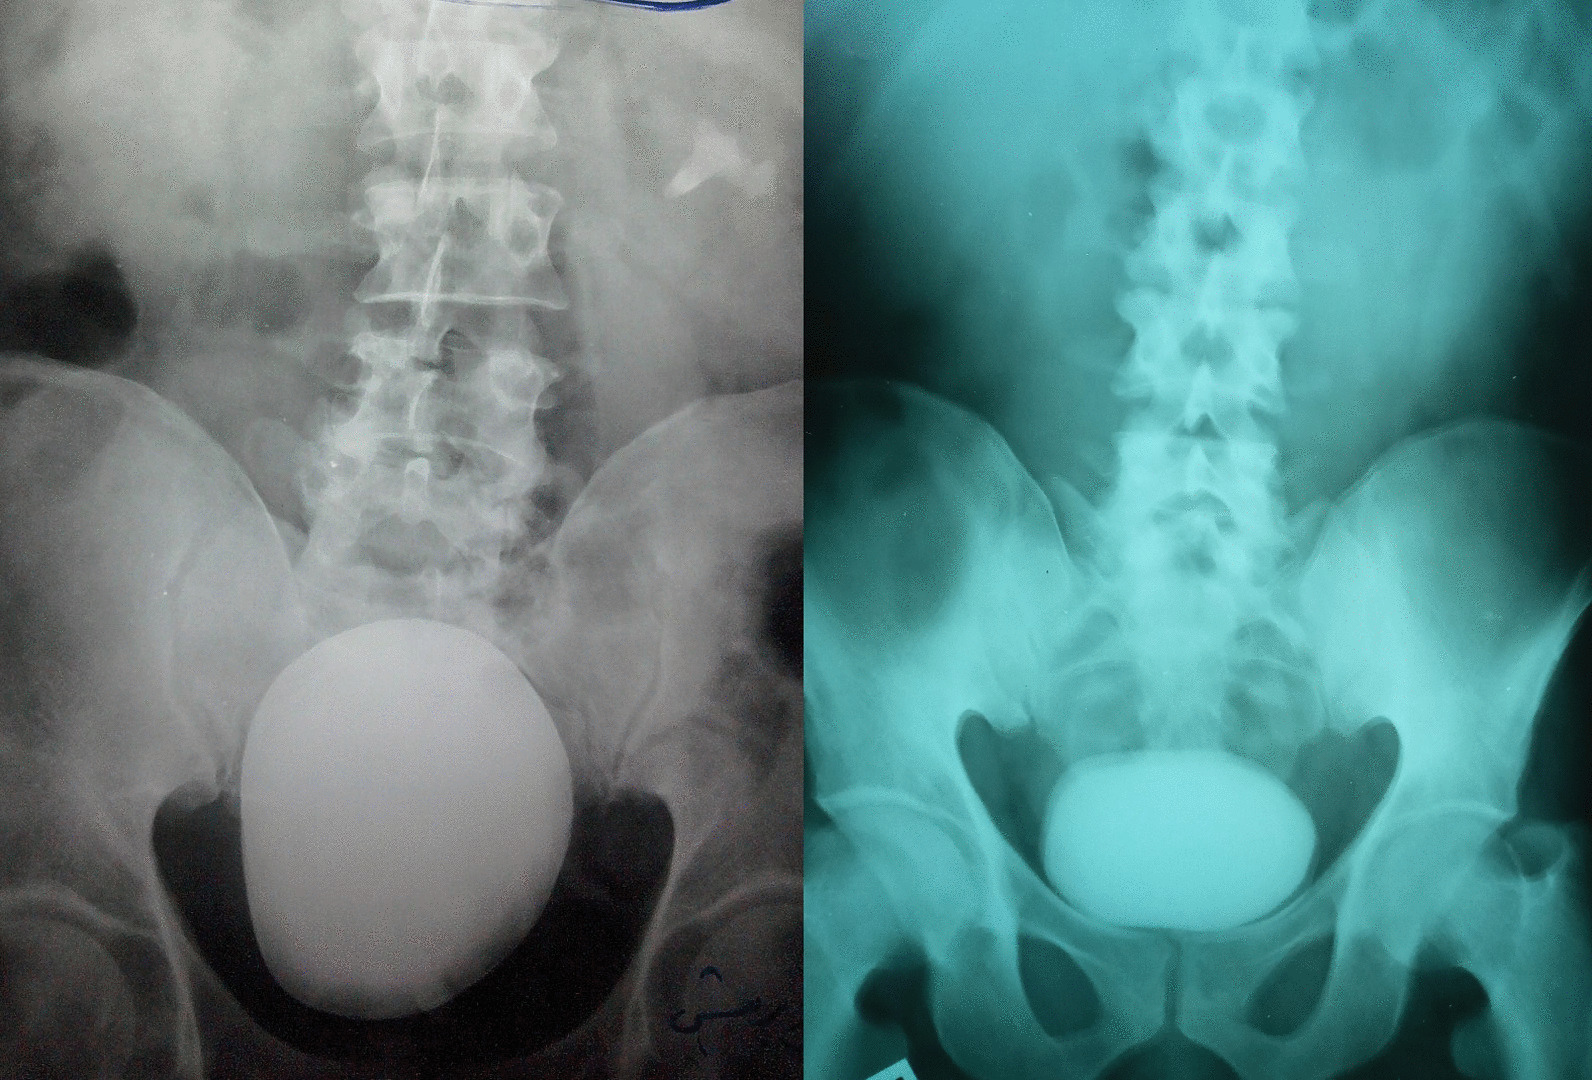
Single huge oval stones with high densities and different lies in plain radiographs: The left-sided figure shows is a huge stone with a vertical lie in a patient with a neobladder and a co-existent branched left kidney stone. The right-sided figure shows a huge stone with a transverse lie and a faint layer on a huge dense core in a native bladder
